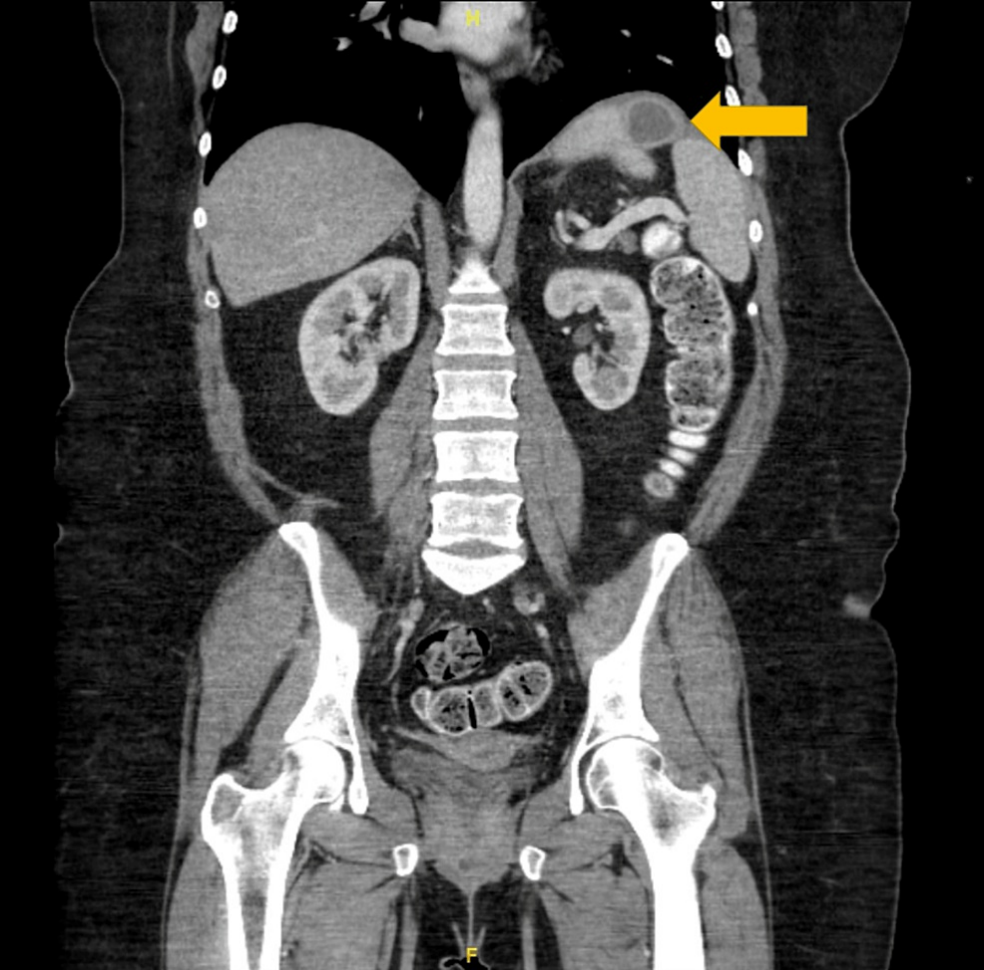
Preoperative CT scan. Coronal CT scan showing sub-diaphragmatic abscess (arrow) secondary to gastropleural fistula.
